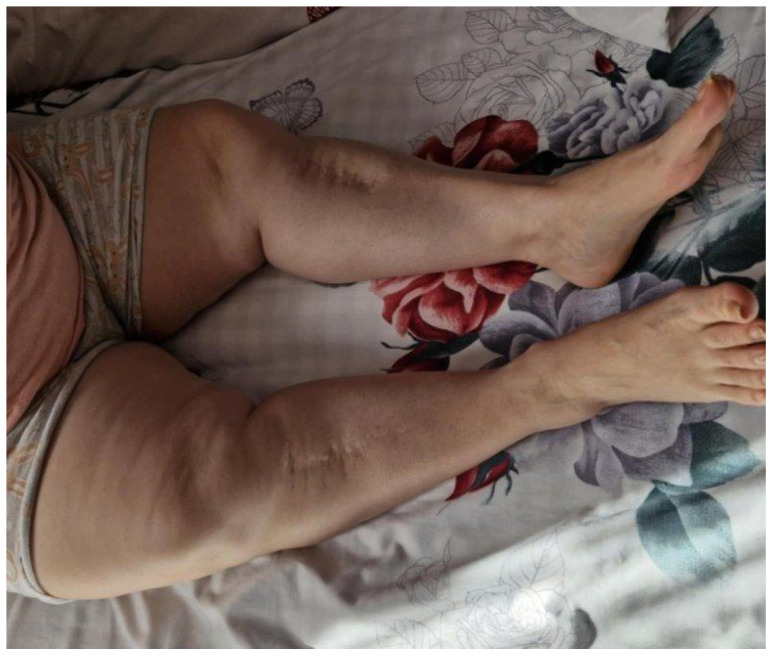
Clinical presentation of patient A.D. (female, 42 years, mother of A.R.) characterized by short stature, moderate genu varum and reduced range of motion at the knees and hips. Oral examination revealed partial edentulism and advanced periodontal disease. Comorbidities were notable. She suffered from severe bilateral coxarthrosis and gonarthrosis, confirmed radiographically. Thyroid ultrasound revealed multinodular goiter with TIRADS 4 nodules in both lobes, requiring surveillance. She also reported progressive left-sided hypoacusis, a hallmark of the disease. Neurological and psychological assessment documented mild cognitive impairment (MMSE 22/30).
